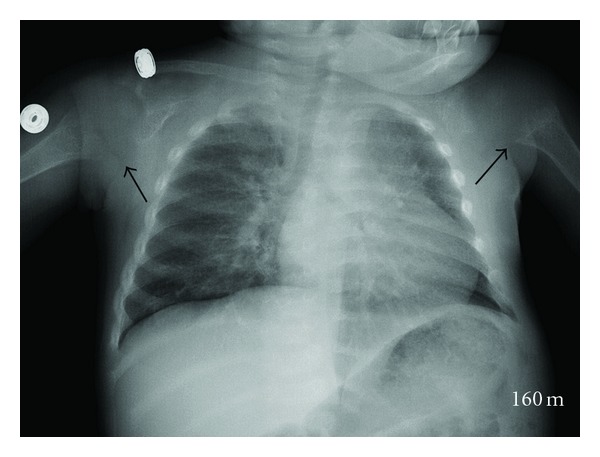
Chest radiograph of a 17-month-old female with pain and recent trauma. The proximal metaphyses of each humerus are irregular with widening (long arrows).
